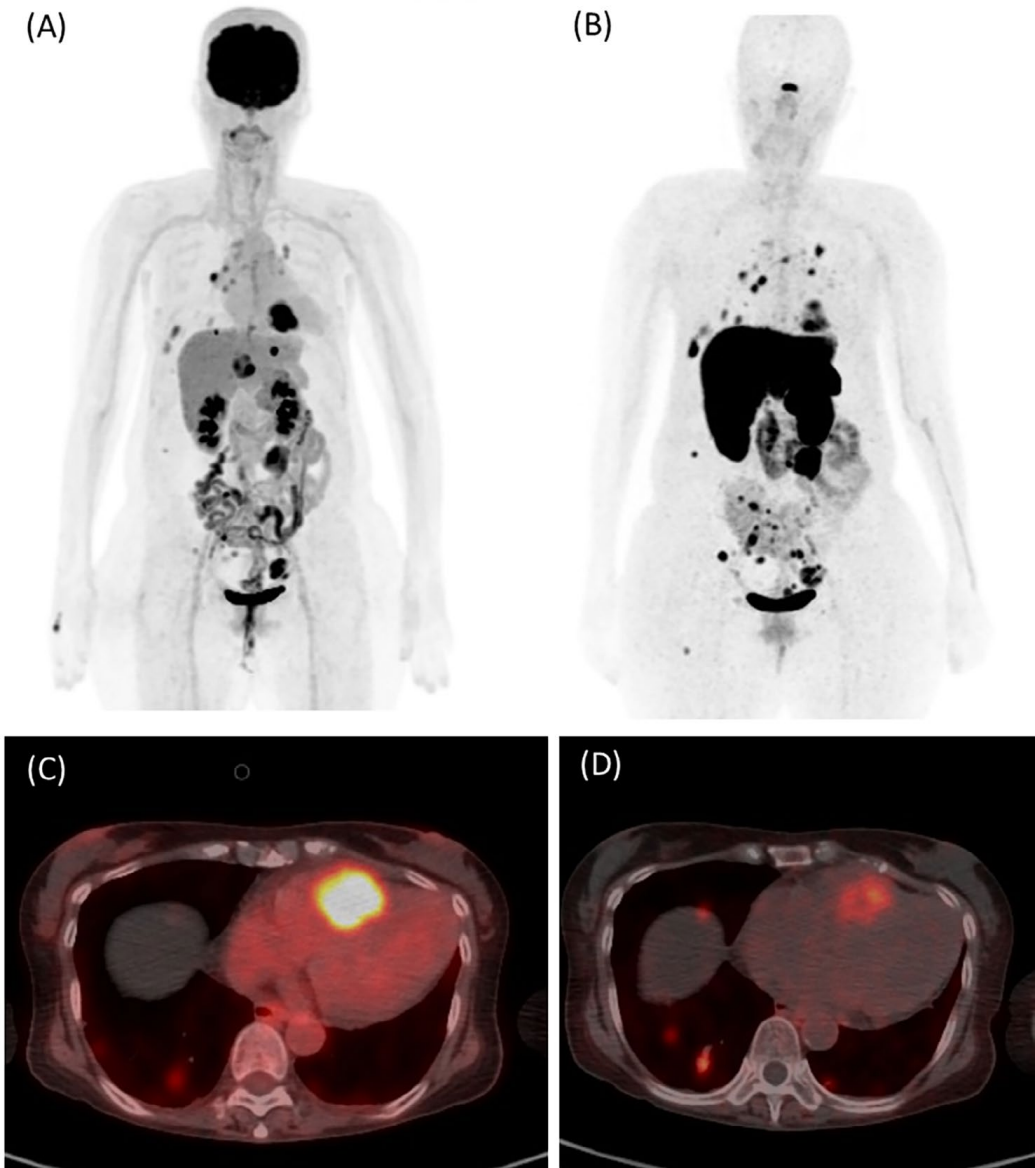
On the maximal intensity projection (MIP) of F‐18 FDG PET (A), hypermetabolic metastatic lesions were observed in both lung fields, the hepatic hilum, a peritoneal mass, intra‐abdominal lymph nodes, and, notably, the heart. In the Ga‐68 DOTATOC PET image (B), multiple lesions with higher uptake compared to FDG PET were identified in both lungs and intra‐abdominal lymphadenopathies. The intracardiac tumor exhibited high FDG uptake (SUVmax 13.46, [C]), while Ga‐68 DOTATOC uptake was mild to moderate (SUVmax 8.36, [D]).
